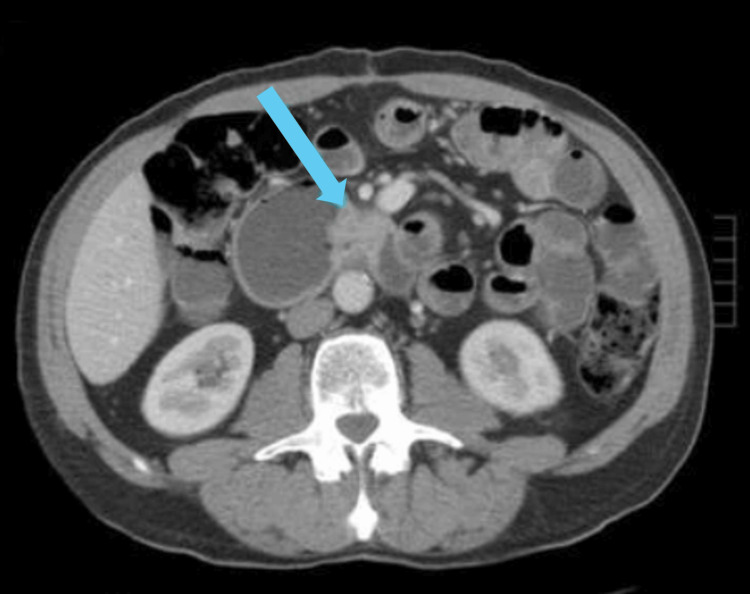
CT scan showing enlarging masses (arrow) in the left upper abdomen, suggesting small bowel lymphoma with adenopathy
